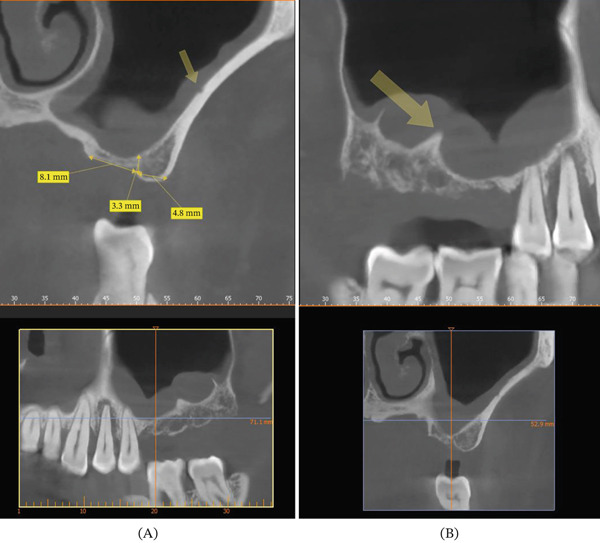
Preoperative CBCT demonstrated that there is a blood vessel in the bone wall of the lateral wall of (A) the maxillary sinus, and there is a septa formation between (B) Teeth #26 and #27.
